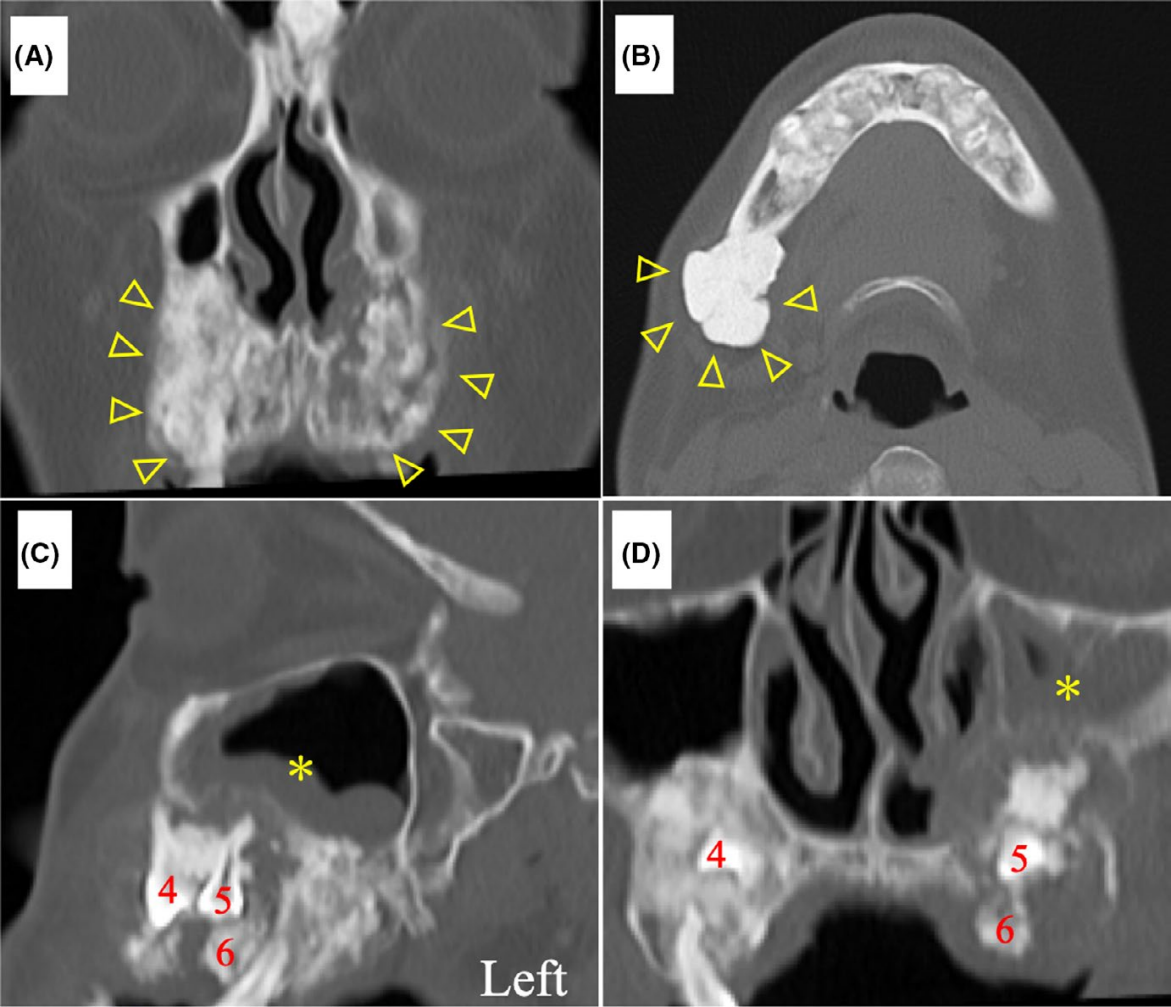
Initial computed tomography image shows nodular osteosclerosis of the maxilla and mandible (A, B; yellow triangles), multiple impacted maxillary teeth (C, D; 4, 5, and 6), soft tissue shadows (*), and bone erosion around the palatal root of the left upper first molar and upper left impacted tooth, which formed a compound odontoma. The soft tissue shadow (*) around the compound odontoma is continuous with the left maxillary sinus (C, D). (4: first premolar, 5: second premolar, 6: first molar)
